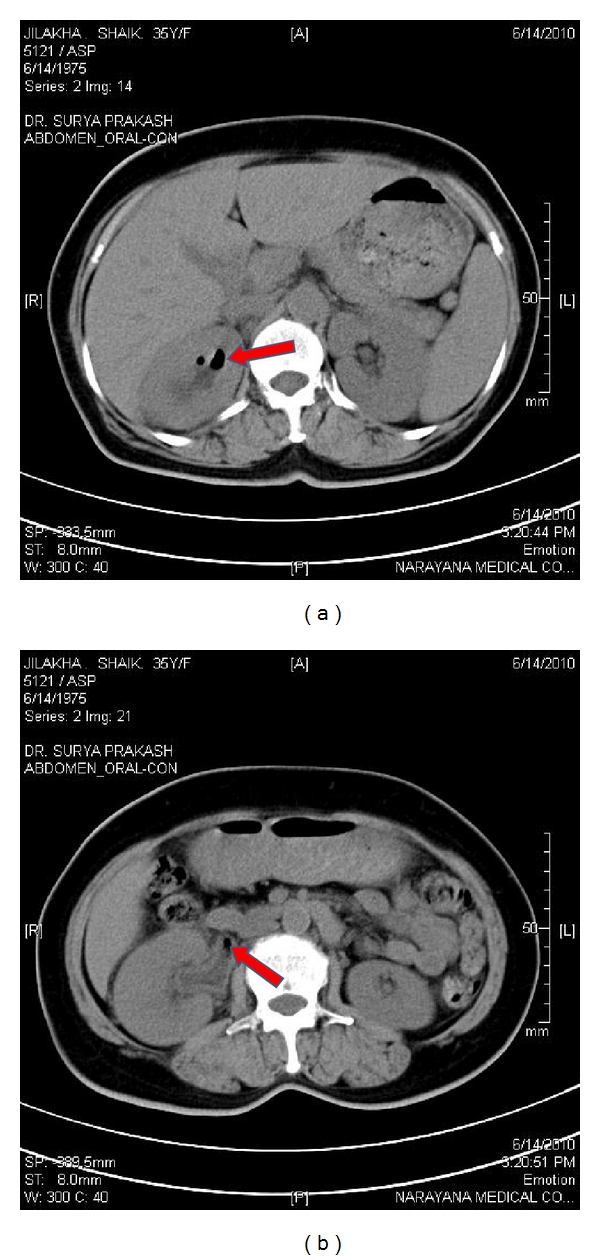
(a) CT KUB showing air pockets in right collecting system. (b) CT KUB showing air pocket in right proximal ureter.
